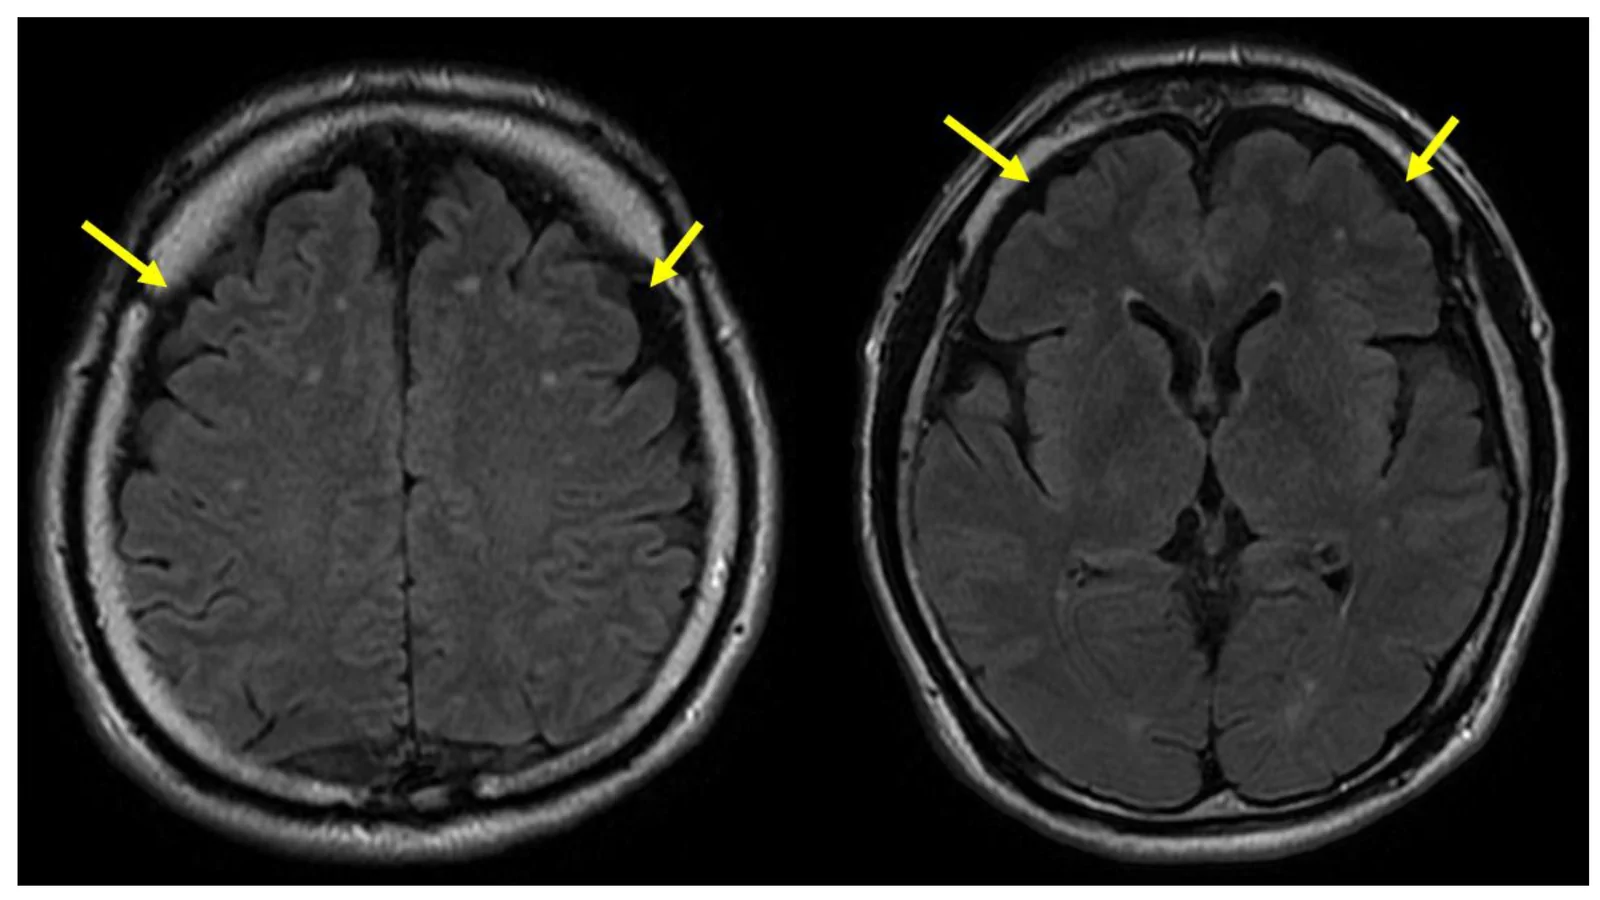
Brain magnetic resonance imaging (MRI). T2-weighted fluid-attenuated inversion recovery (FLAIR) images showing mild atrophy in the bilateral frontal lobes (yellow arrows).
